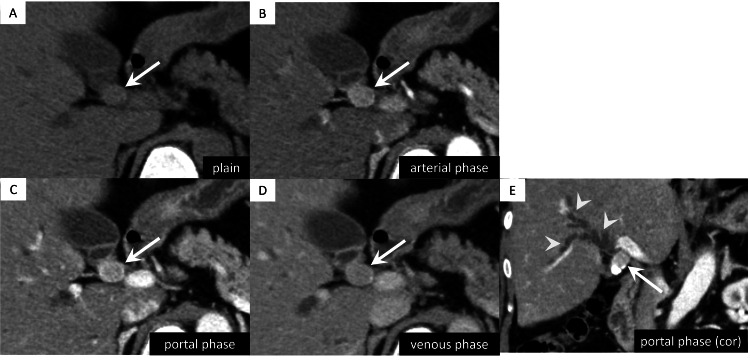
Abdominal computed tomography (CT) imaging findings. (A) Non-contrast CT showing a 17 mm × 15 mm × 13 mm nodule (arrow) at the confluence of the three bile ducts.
